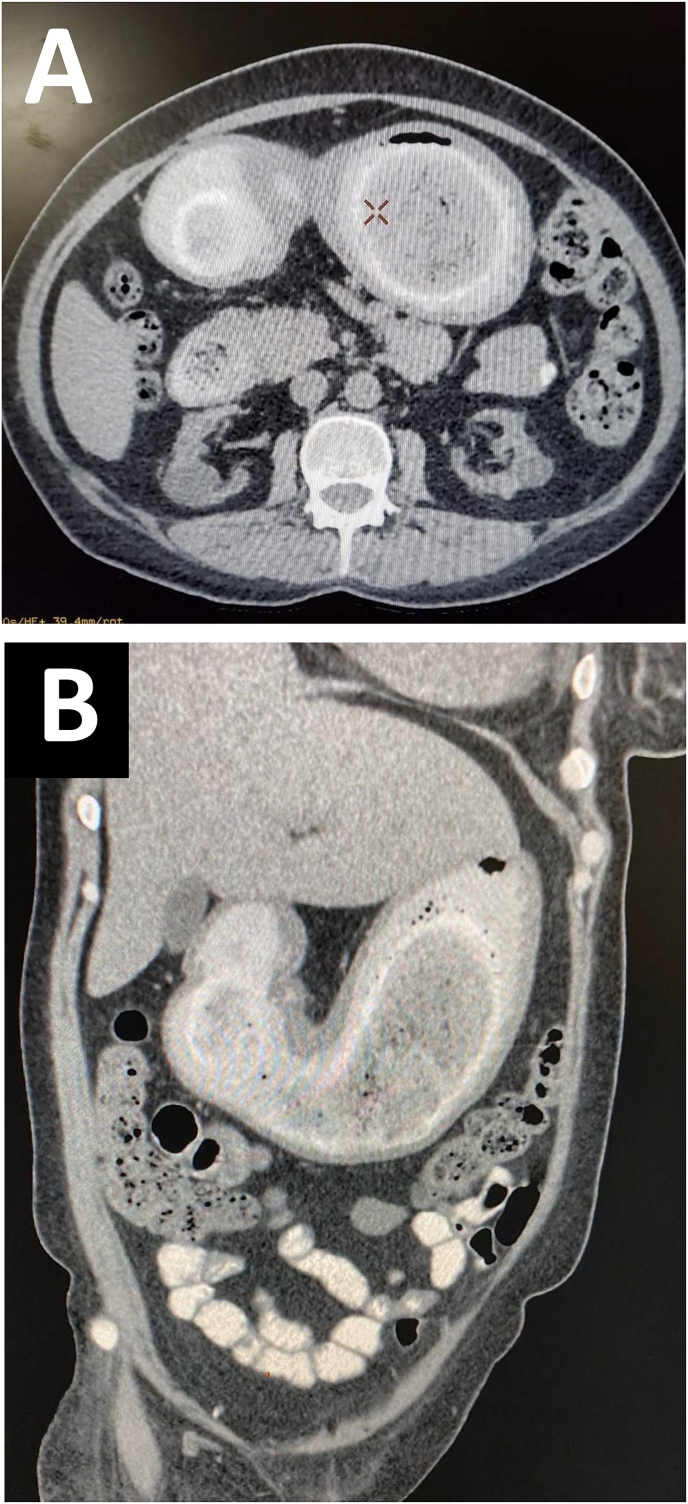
CT at axial (A) and coronal view (B) showed a bezoar in the stomach extending to the second part of the duodenum as outlined by contrast.
